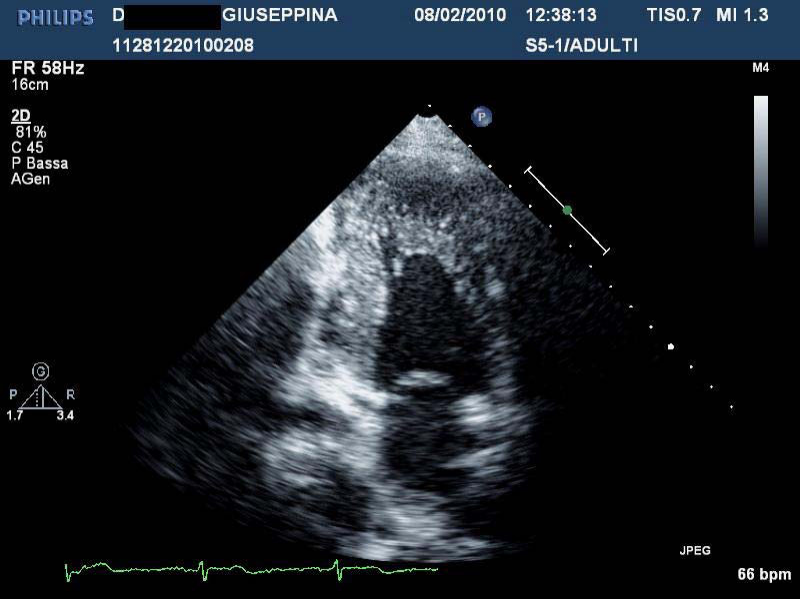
Patient with left ventricular non compaction. This patient was sottoposed to echocardiography in the contest of family screening for apical hypertrophic cardiomyopathy.
